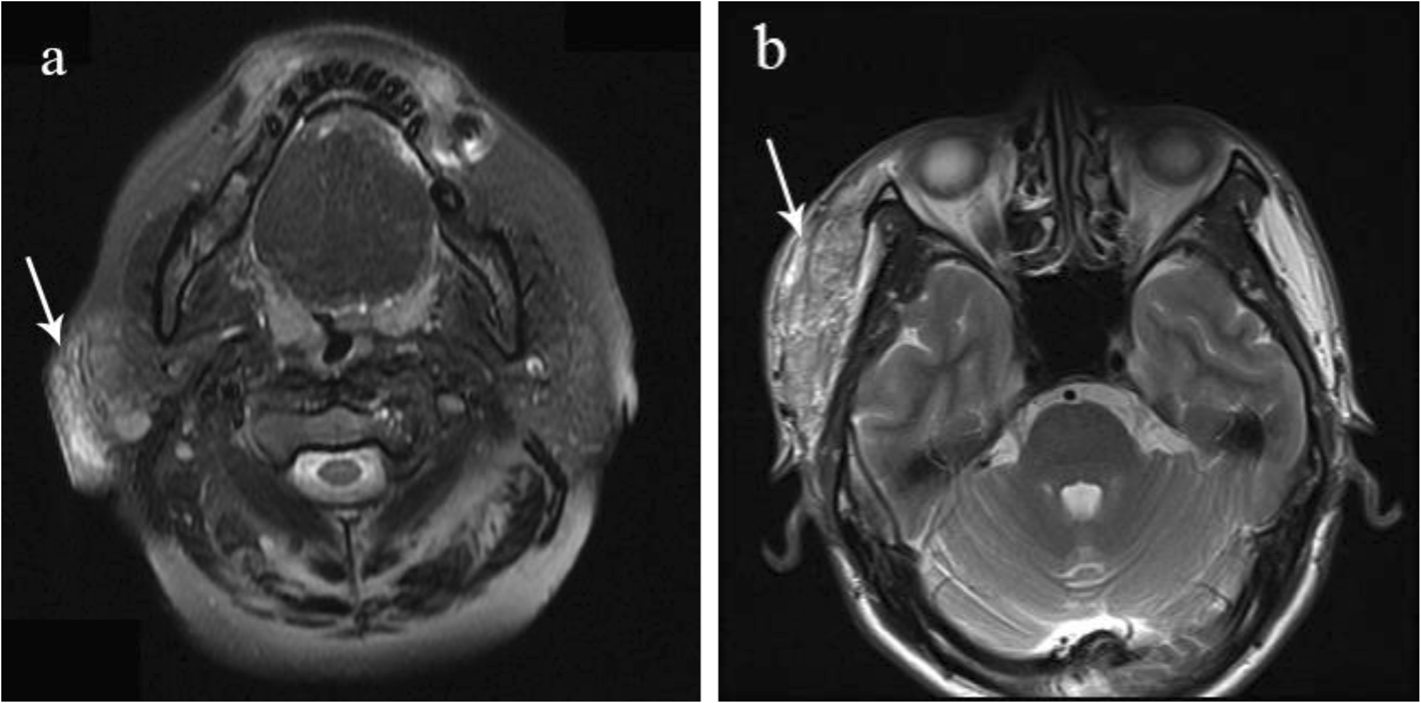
Mass was hyper-intense on axial T2-weighted image on two patients (arrow). a A 51-year-old female patient presented with one-year history of a pruritus mass in the right parotid region; b A 44-year-old male patient presented with one-year history of a mass with melanin pigmentation in the right temporal region
